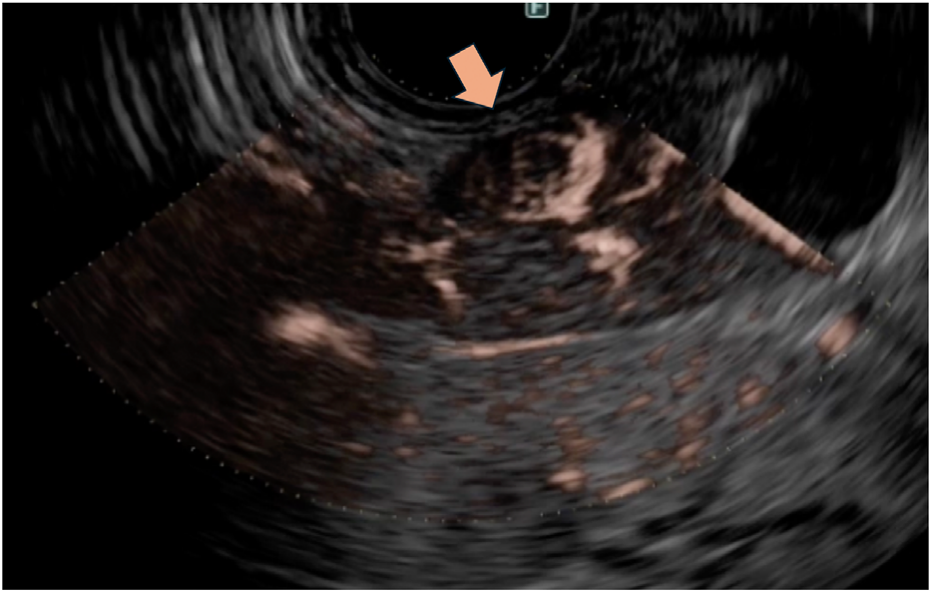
Detective flow imaging endoscopic ultrasound was able to visualize a small 8.9 mm tumor in the pancreatic tail (arrow) by depicting tumor vessels characteristic of pancreatic neuroendocrine tumors.
